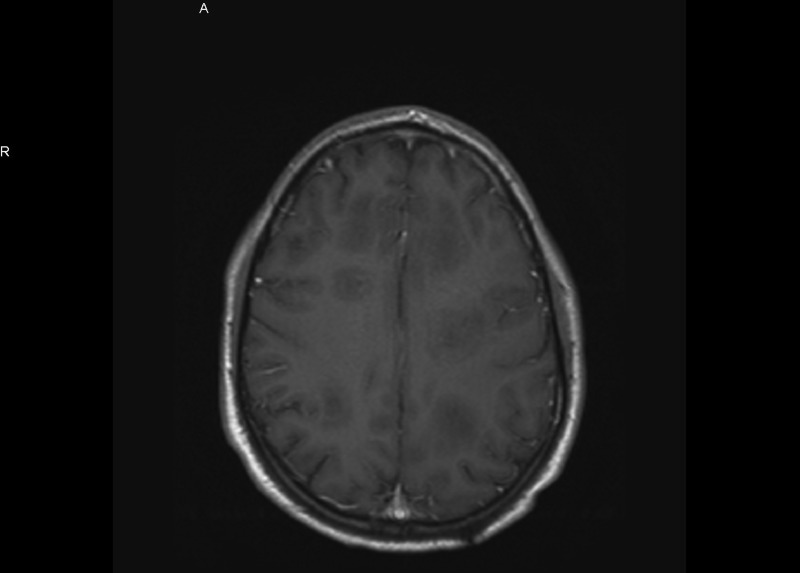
MRI without contrast after corticosteroid treatment shows increased size of the lesions.
